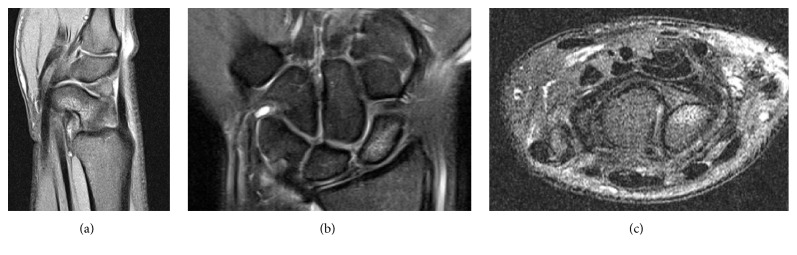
(a) A 1.5-Tesla MRI T2 sagittal cut demonstrating palmar scaphoid waist bone edema consistent with incomplete scaphoid waist stress fracture obtained at the time of initial presentation. (b) A 1.5-Tesla MRI T2 coronal cut showing scaphoid waist bone edema consistent with incomplete scaphoid waist stress fracture obtained at the time of initial presentation. (c) A 1.5-Tesla MRI T2 axial cut showing palmar scaphoid waist bone edema consistent with incomplete scaphoid waist stress fracture obtained at the time of initial presentation.
